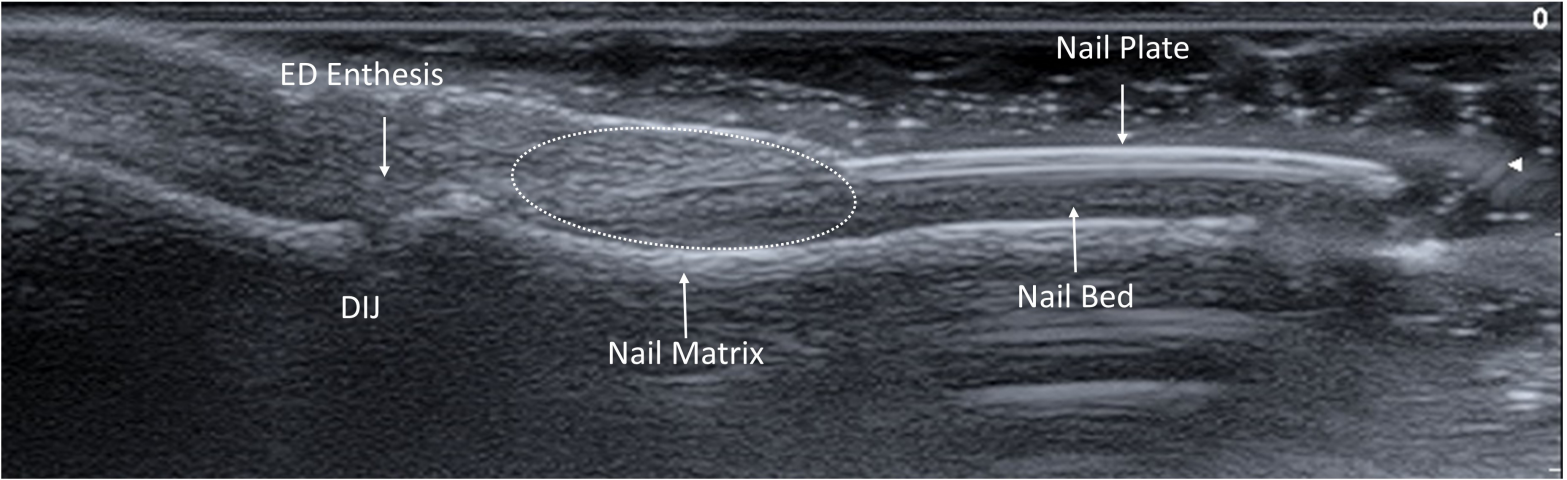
Ultrasound anatomy of the nail-enthesis complex. The nail-enthesis complex is made of multiple structures. The extensor digitorum ED tendon merges with the DIJ capsule and further branches into two components. The deep fibers attach to the bone cortex of the distal phalanx, while the superficial fibers represent the supporting scaffold of the nail matrix, from which the nail plate develops and extends distally to for a trilaminar structure with two linear hyperechoic bands split by a hypoechoic one. The nail plate is held in place by the nail bed that connects it with the bone cortex of the distal phalanx. ED, Extensor Digitorum; DIJ, Distal Interphalangeal Joint.
